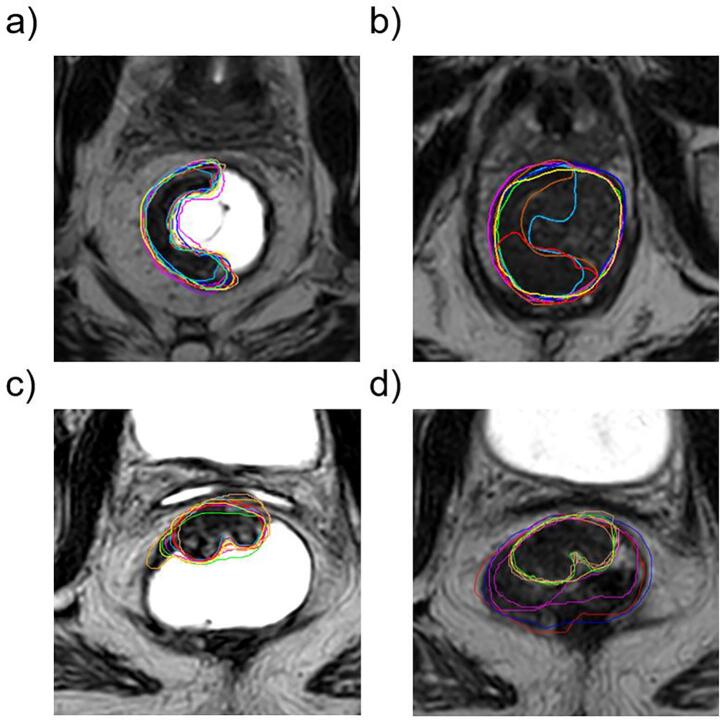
Representative examples of delineations returned by observers for case 2 (a) and b)) and case 6 (c) and d)). Sections a) and c) correspond to T2 MRI sequences with 100 cc of ultrasound gel applied prior to the scan and b) and d) to scans without the ultrasound gel.
